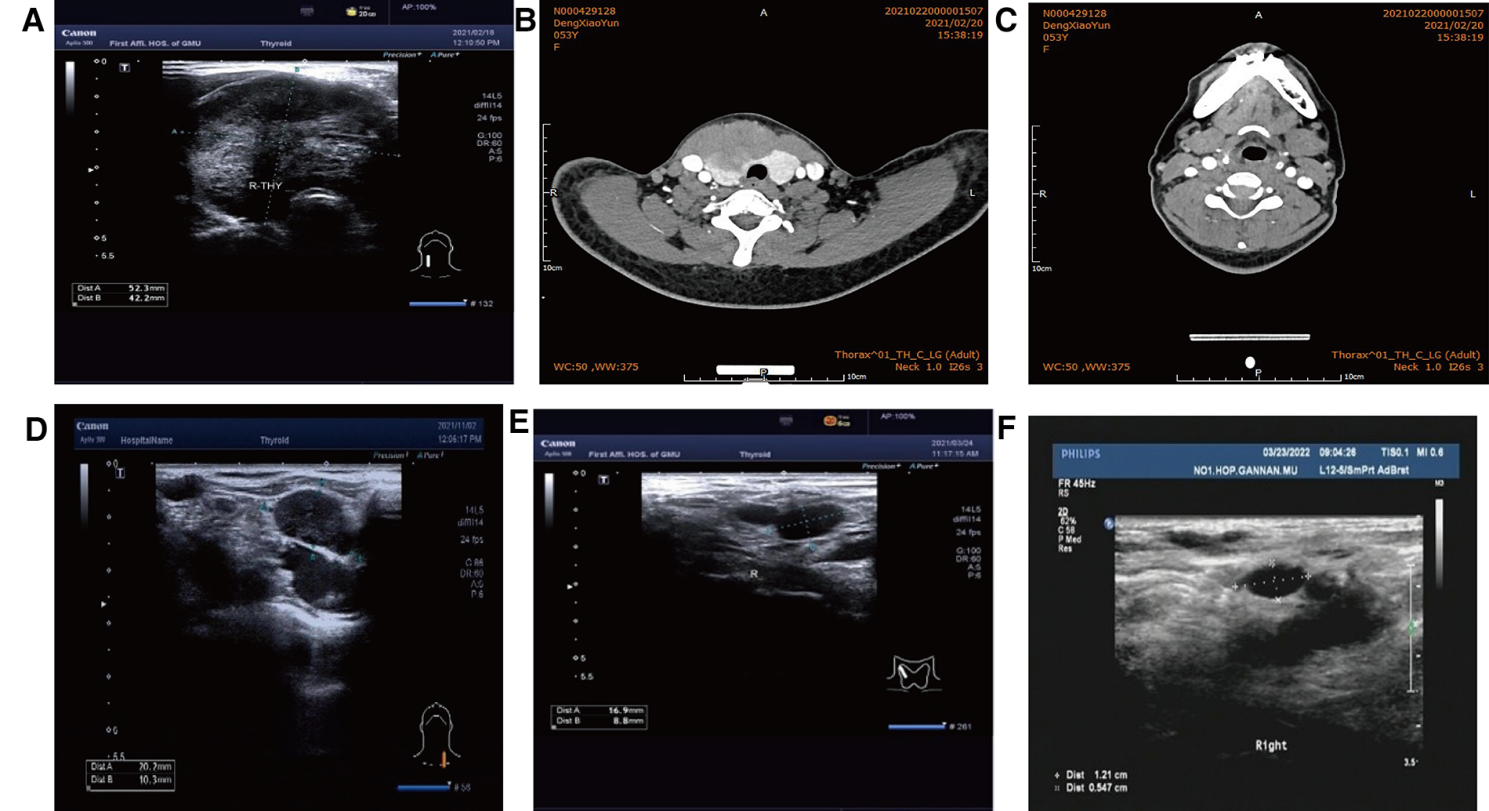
(A) Color ultrasound (52 mm × 42 mm hypoecho was seen in the right lobe of the thyroid gland, occupying almost the whole right lobe, with clear boundary and blood flow signals visible inside). (B) CT (irregular low-density mass, about 61 mm × 40 mm in size, unclear boundary, moderately uneven enhancement on enhanced scan). (C) Postoperative cervical CT. (D) Before radiotherapy. (E) 1 month after radiotherapy. (F) Lymph node shrinkage after local radiotherapy was observed by lymph node color ultrasonography 6 months after radiotherapy.
